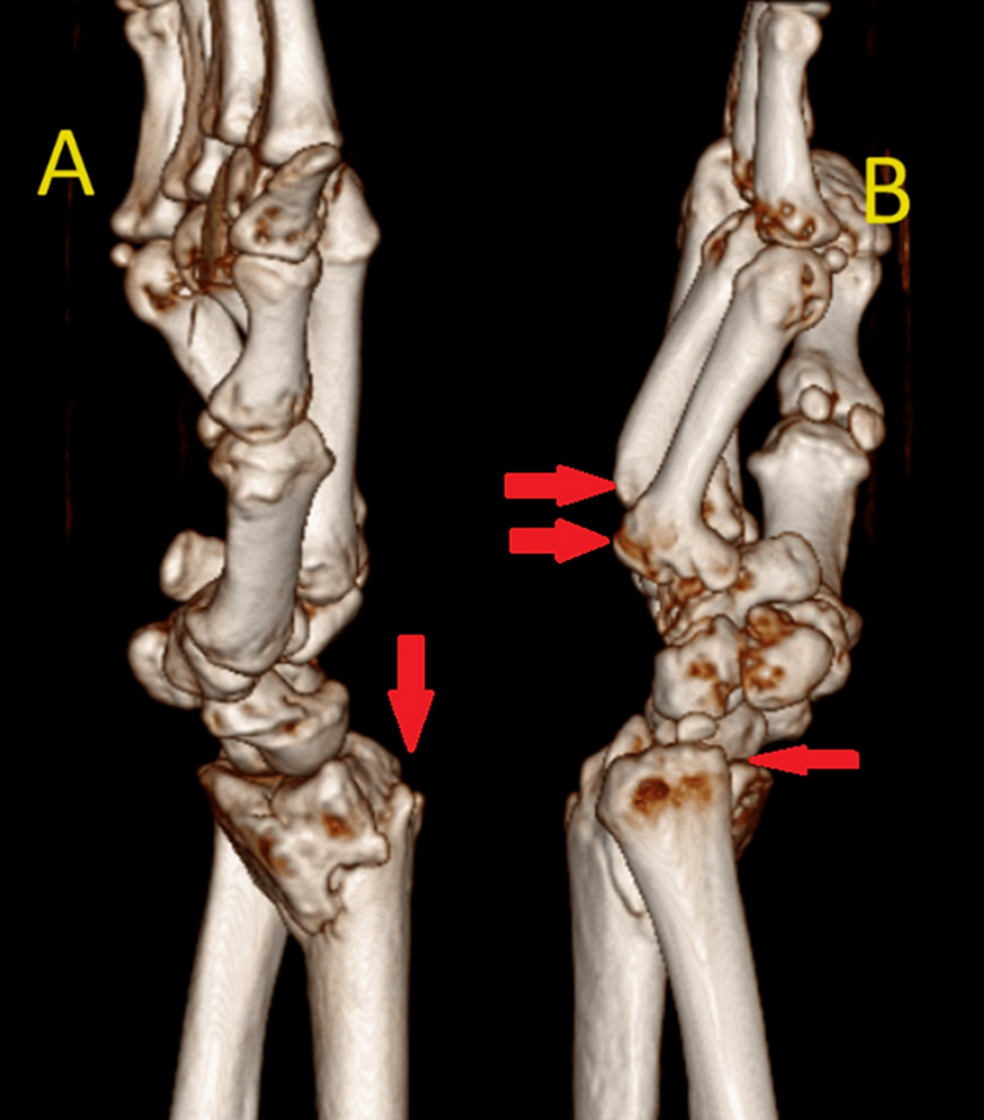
Computed tomography (CT) scan and 3-dimensional reconstruction image of the right wrist with hand lateral (A) and medial (B) views (A, B) show the malunited distal end radius fracture and the malunited base of the 4^th^ metacarpal fracture on the right side.
